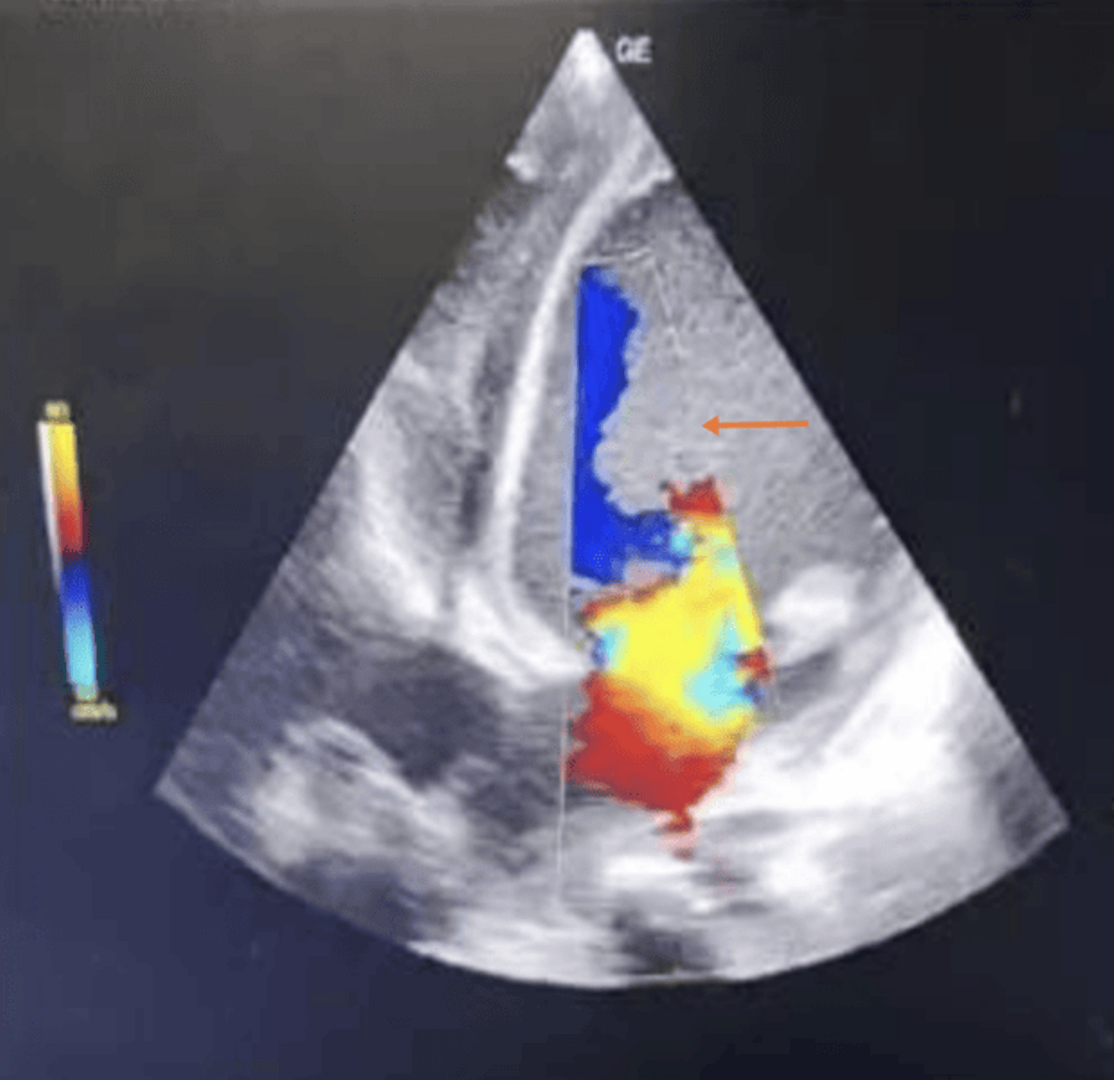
Echocardiography shows left ventricular dilatation with mitral regurgitation in a case of dilated cardiomyopathy
